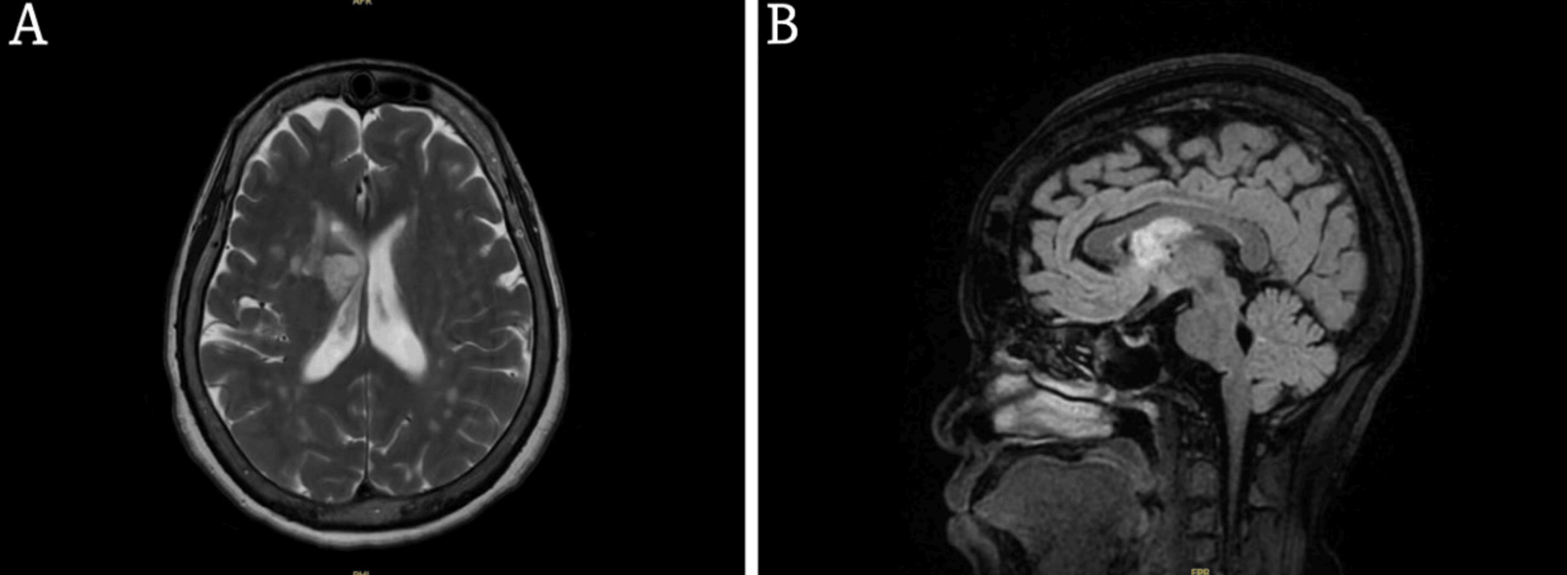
A and B. MRI T2 transverse and sagittal views showing a lesion centered within the right lentiform nucleus with associated multifocal peripheral enhancement.
